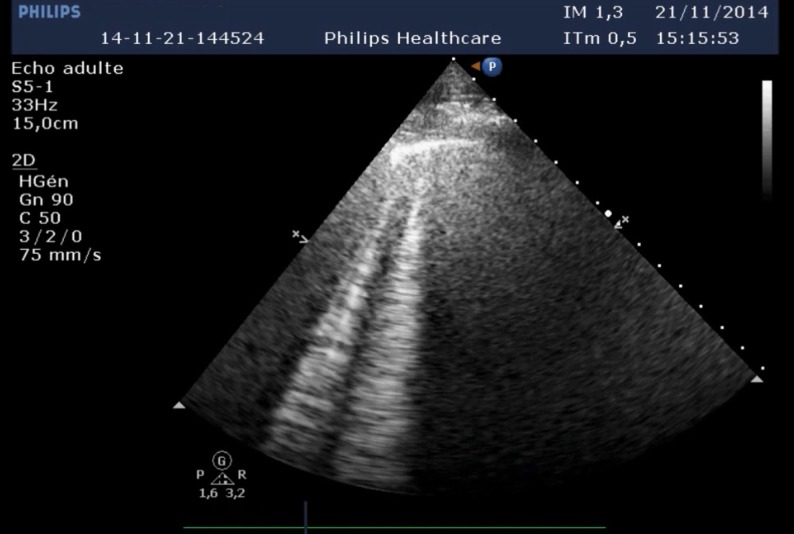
Example of comet pictures visualized by transthoracic echocardiography in a patient hospitalized
